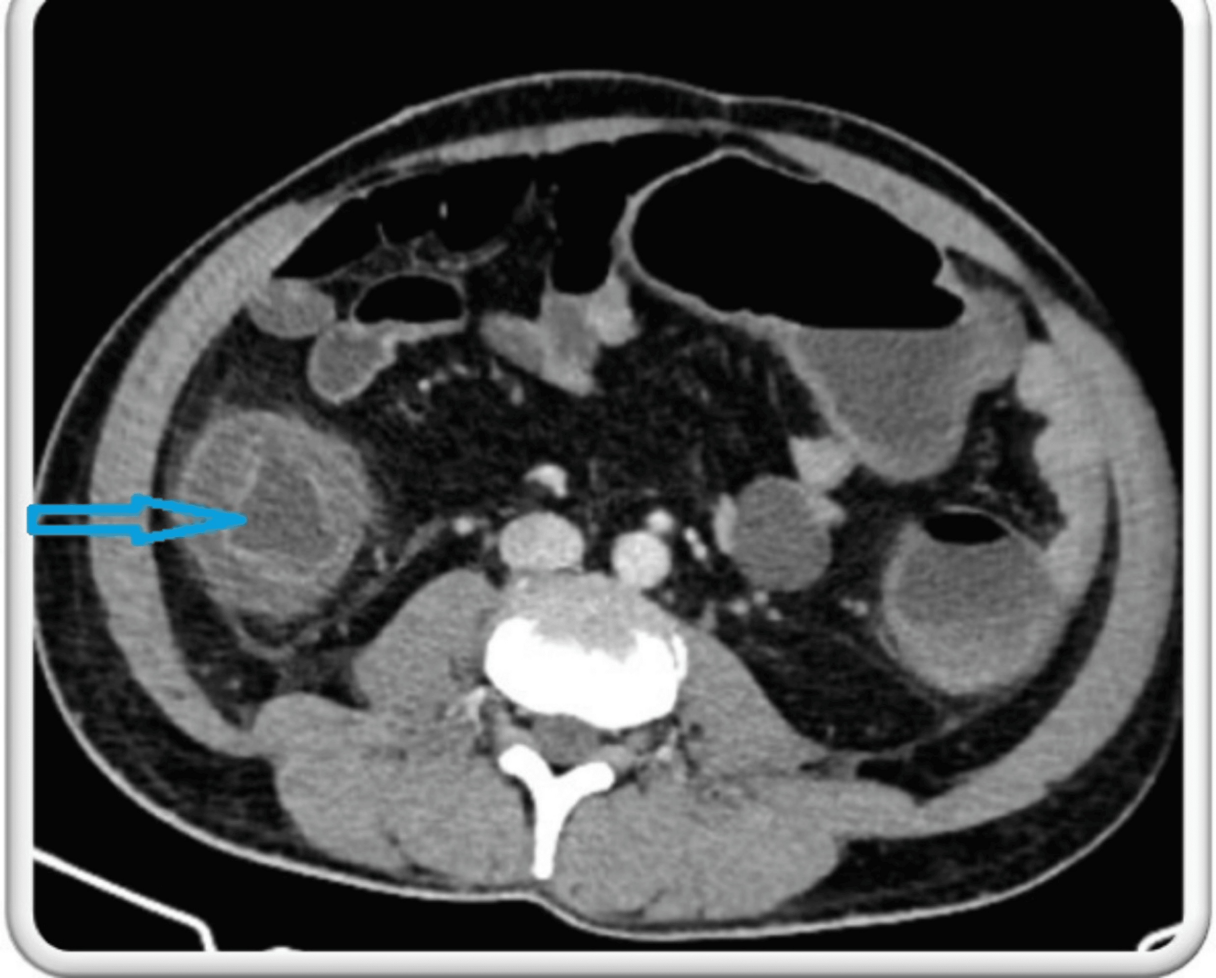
CECT of the abdomen The blue arrow shows diffuse symmetrical smooth circumferential mural wall-thickening with submucosal edema (water target sign) involving the entire colon, suggestive of pancolitis CECT: contrast-enhanced computed tomography
